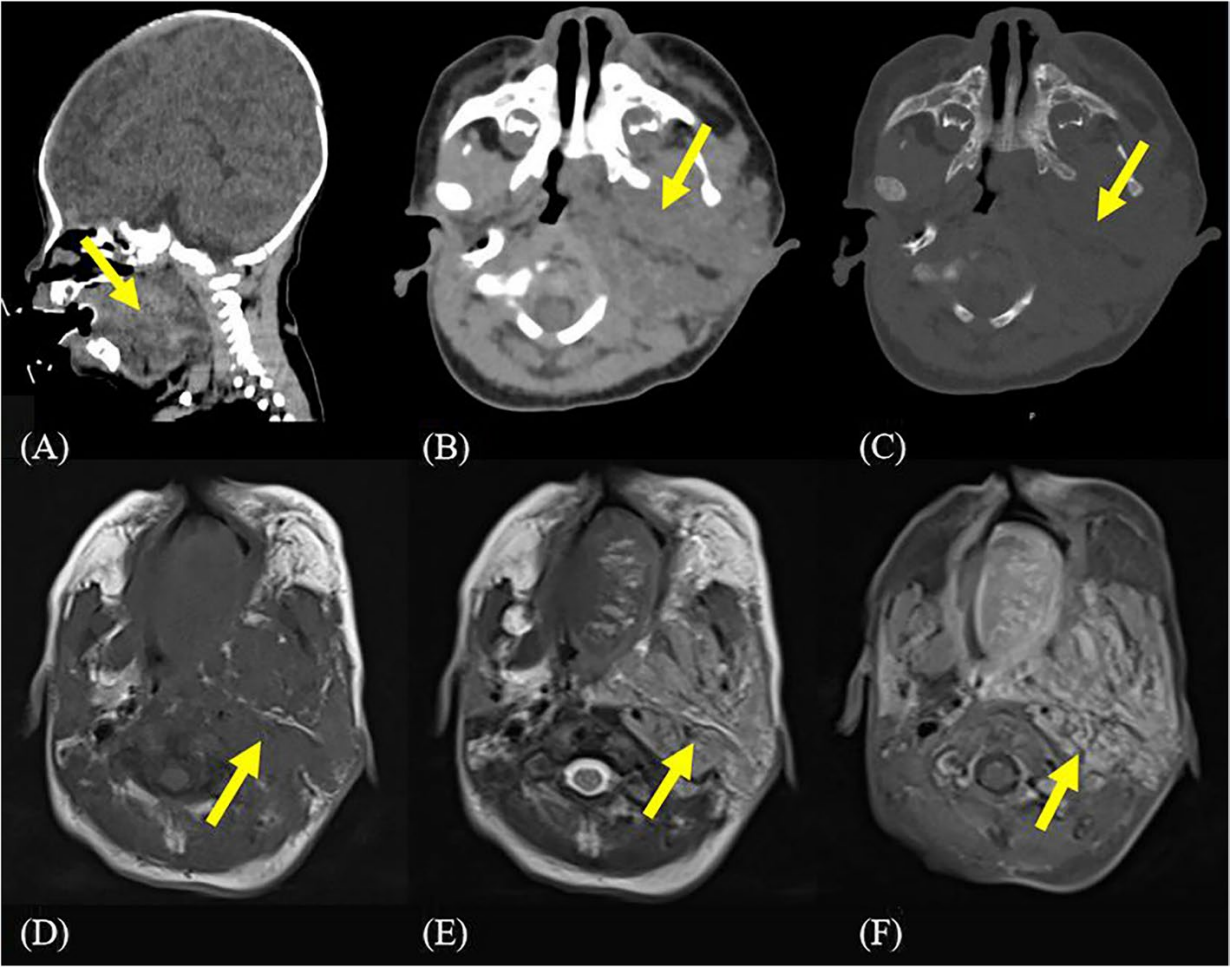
CT & MR images. A Sagittal soft tissue window shows a large mass occupying in the left maxillofacial and cervical region (arrow); B Coronal soft tissue window shows that the lesion involves the left parapharyngeal space (arrow); C Coronal bone window shows localized bone destruction in the left mandible (arrow); D Enormous mass occupying in the left maxillofacial and cervical region involves soft tissues such as the parapharyngeal space, with mixed iso-signal in T1-weighted imaging (arrow) and E mixed high signals in T2-weighted imaging (arrow); F Contrast enhanced MRI shows significantly more uniform enhancement, partly nodular and lumpy enhancement (arrow)
